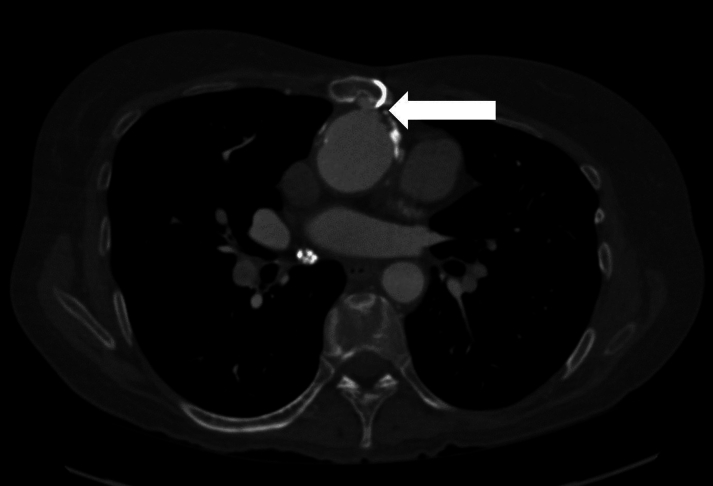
Computed Tomography Scan Demonstrating an Aortic Pseudoaneurysm Invading the Sternum
